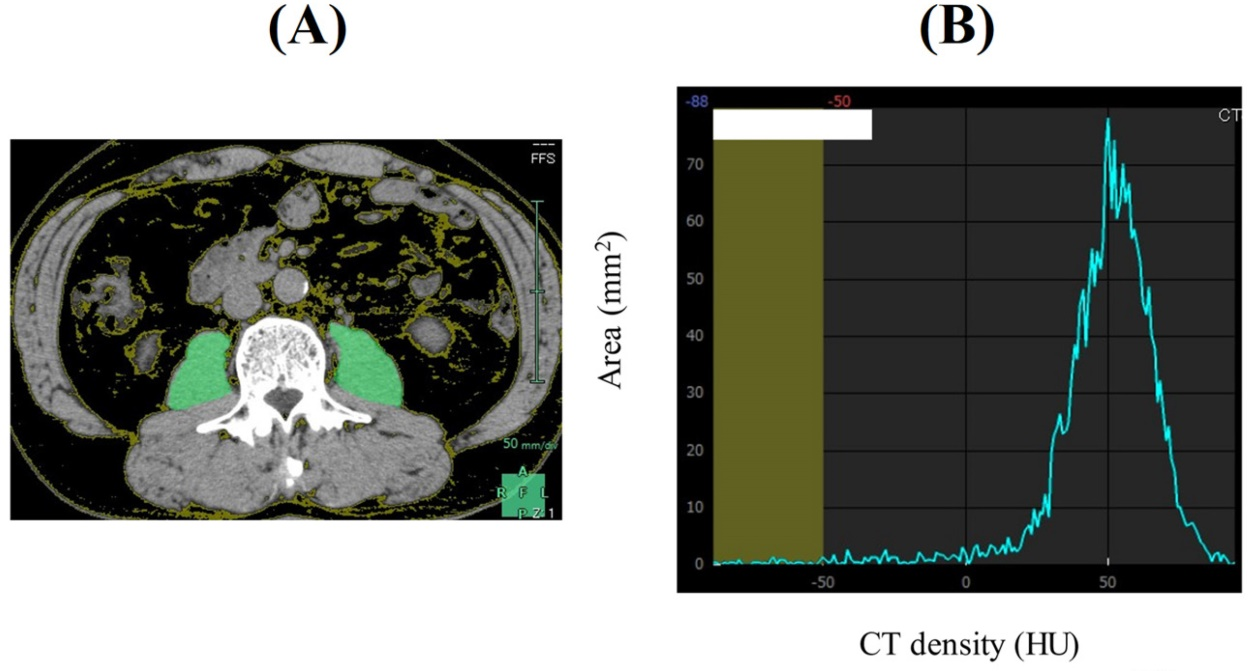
A representative CT image at the lumbar three level (Figure 1A). The sum of the areas within the left and right psoas muscles corresponding to fat (cm^2^, CT density: -200 HU to -50 HU) was calculated by Vincent®. In this case, area under the curve corresponding to CT density less than -50 HU is 13.71 mm^2^. The whole area under the curve is 2207.15 mm^2^. Thus, FRPM is calculated as 0.67% (Figure 1B).
